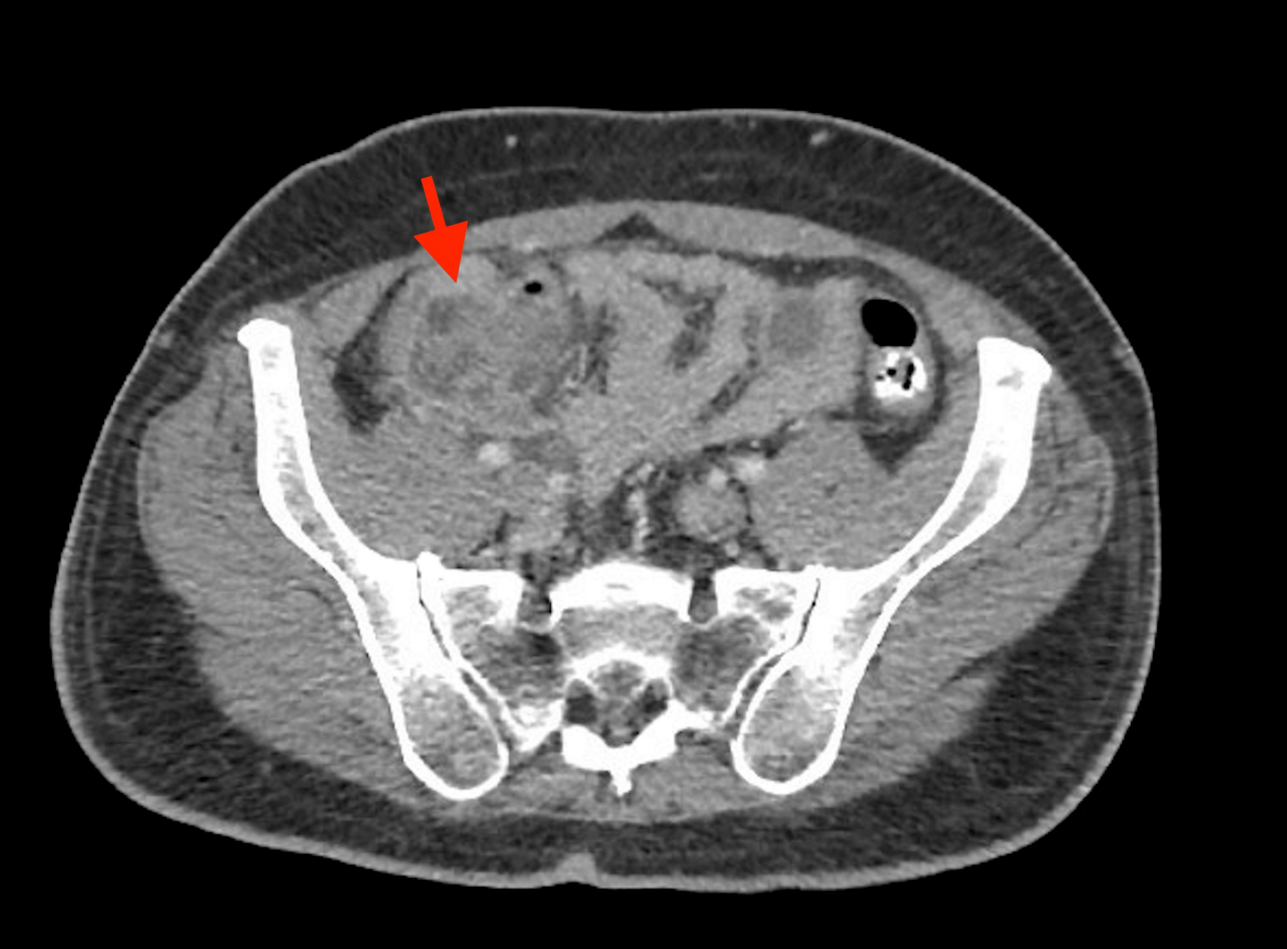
CT abdomen (axial view) findings showing appendiceal thickening w/ surrounding inflammation in RLQ consistent with appendicitis CT: computed tomography, RLQ: right lower quadrant
